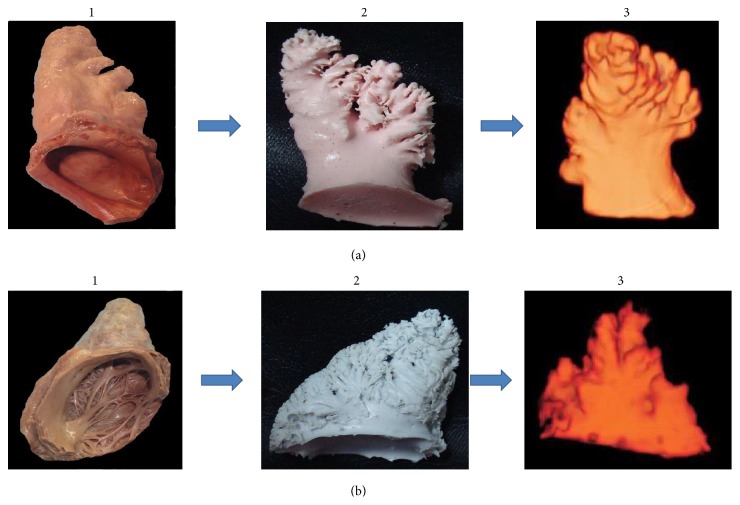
Representative molds of the right atrial appendage (RAA) and the left atrial appendage (LAA). (a)-1 LAA was dissected from the donated body. (a)-2 A silicon mold of LAA. (a)-3 Multidetector computed tomography (MDCT) scanning image of mold of LAA. (b)-1 RAA was dissected from the donated body. (b)-2 A silicon mold of RAA. (b)-3 MDCT scanning image of mold of RAA.
